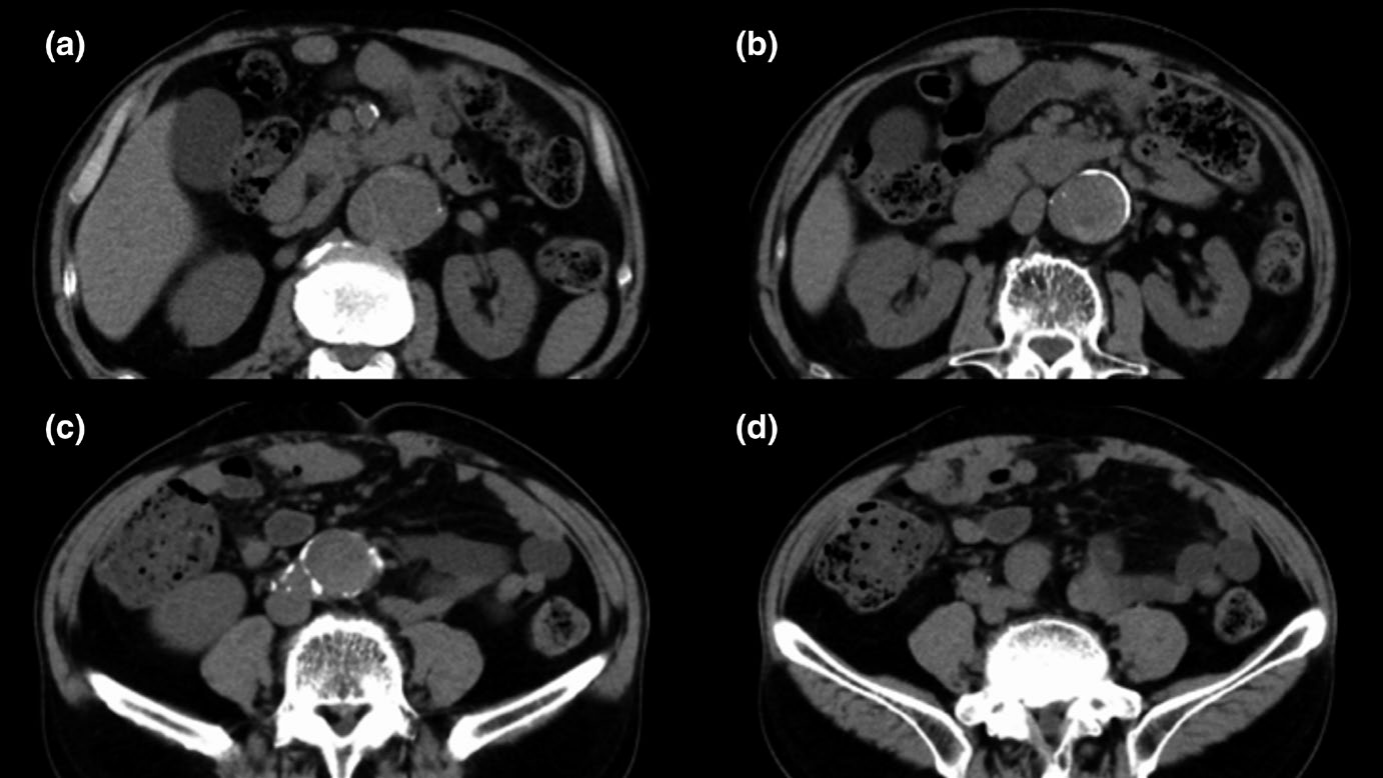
A computed tomography angiogram does not show aortic dilatation. The dissection in the thoracoabdominal aortic lesions has a maximum diameter of 38 mm. (A) At the level of the superior mesenteric artery, (B) at the level of the renal arteries, (C) infrarenal level, and (d) at the level of the terminal aorta
